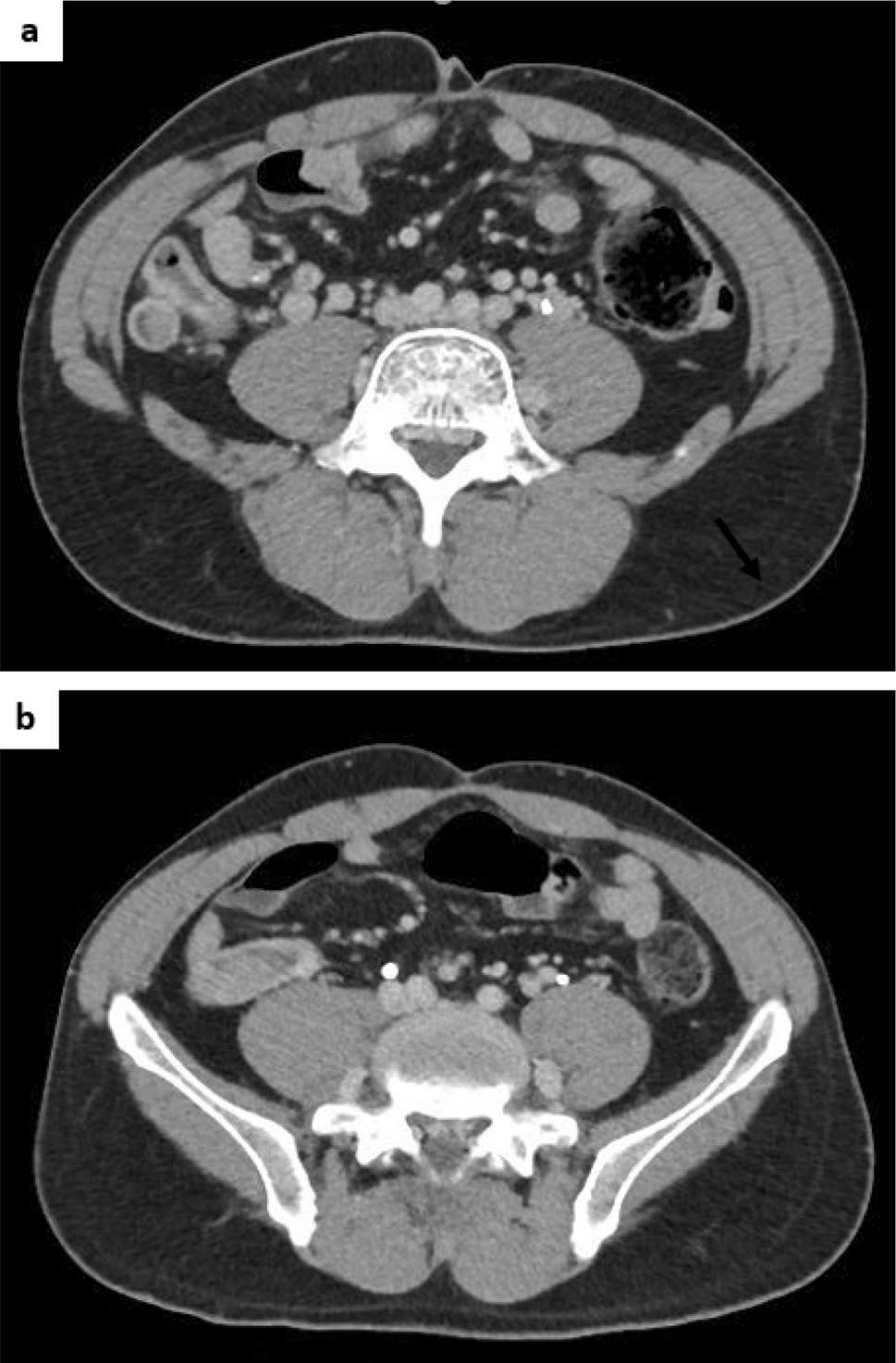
Abdominal contrast computed tomography performed in February 2017. a, b An enlarged appendix is shown
